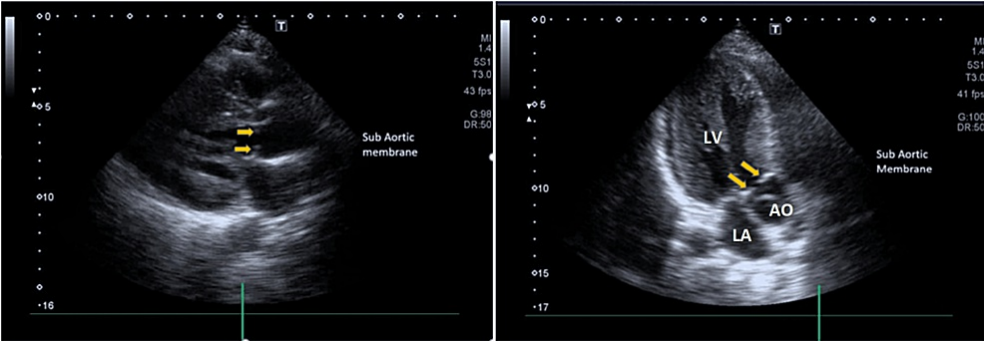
PLAX and APLAX view 2-D imaging showing severe LVH, septum is relatively thicker as compared to posterior wall (ASH). There is a sub-valvular membrane. Figure 2 (Left): 2-D PLAX in a diastolic frame (as the mitral valve is slightly open) showing severe LVH. The septum is relatively thicker as compared to posterior wall. Arrows pointing towards sub-aortic membrane. Figure 2 (Right): 2-D APLAX (three chamber) view in a systolic frame (as the mitral valve is closed and the aortic valve is slightly open). Arrows pointing towards echo dense sub-aortic valve membrane. PLAX=parasternal long axis; APLAX: apical long axis; 2-D: two-dimensional; LVH=left ventricular hypertrophy; ASH=asymmetric septal hypertrophy; LV=left ventricle; LA=left atrium; AO=aorta
